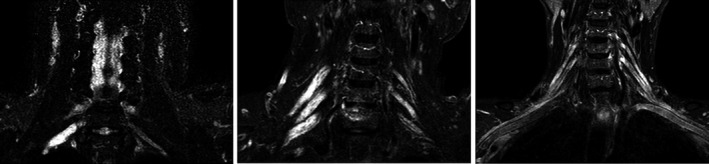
Hypertrophy and nerve thickening on bilateral coronal T2 STIR MR imaging of the brachial plexus in inflammatory neuropathies. Left: unilateral hypertrophy and thickening of cervical nerve roots in an multifocal motor neuropathy (MMN) patient. Middle: Bilateral and asymmetrical abnormalities in an Lewis‐Sumner syndrome (LSS) patient. Right: Bilateral and symmetrical abnormalities in a chronic inflammatory demyelinating polyradiculoneuropathy (CIDP) patient
